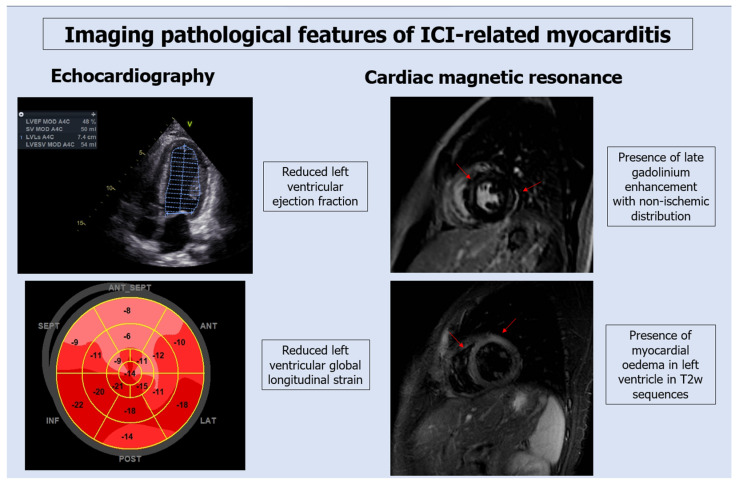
Echocardiographic and magnetic resonance imaging in a patient with ICI-related myocarditis. Echocardiography shows an impaired left ventricular ejection fraction with a reduction in global longitudinal strain with a non-coronary distribution. Magnetic resonance shows presence of late gadolinium enhancement (red arrows in top right figure) in inversion recovery gradient echo (IRGE) sequences and myocardial edema (red arrows in bottom right figure) in T2-weighted sequences.
